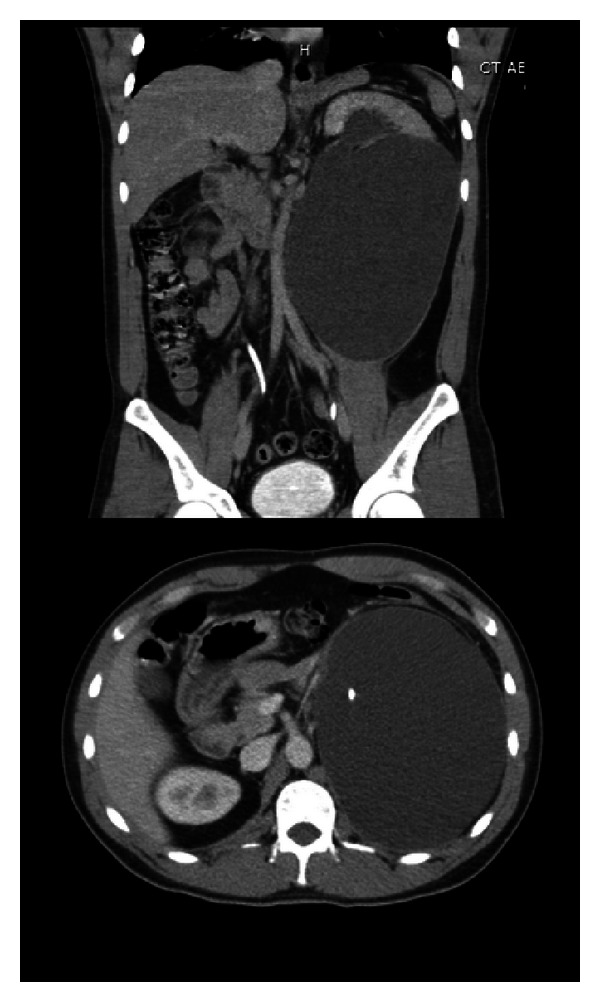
Urinoma seen on repeat CT abdomen and pelvis with contrast after placement of ureteral stent.
